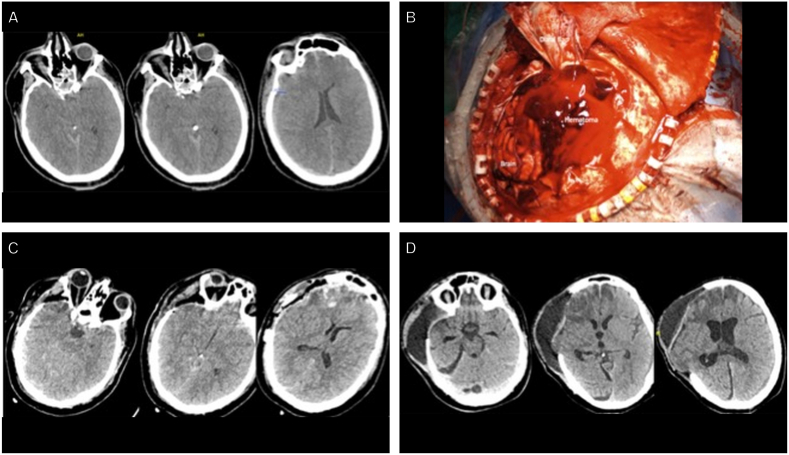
Serial neuroimaging and operative findings. (a) Admission non-contrast CT head showing right frontotemporoparietal acute subdural haematoma with cerebral edema, obliterated basal cisterns, and subarachnoid haemorrhage; Rotterdam CT score 5. (b) Intraoperative photograph after right frontotemporoparietal decompressive craniectomy showing swollen brain with overlying subdural haematoma. (c) Early postoperative bedside CT showing adequate decompressive craniectomy, resolving mass effect and midline shift, and evolving right basifrontal contusion. (d) CT head at 3 weeks before aeromedical transfer showing open basal cisterns, further resolution of mass effect, persistent craniectomy defect, and subgaleal hygroma.
